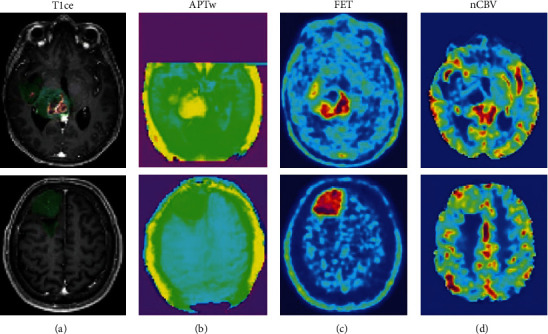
FET PET combined with amide proton transfer (APT) CEST MRI. Example images of a glioblastoma (upper row) and low-grade glioma (lower row). Segmentation (contrast-enhancing tumor (CET) in red, FLAIR hyperintense tumor (FHT) in green) is overlaid on the contrast-enhanced T1-weighted image (a) to show the spatial overlap. FHT in glioblastoma; both amide proton transfer-weighted (APTw) and CBV were significantly (*P* < 0.001, respectively) higher in PET-positive areas. Even in low-grade gliomas, a similar trend was observed for the two imaging modalities (*P* = 0.085 for APTw and *P* = 0.045 for CBV). Reproduced with permission from Figure 2 of Schon et al. [60].
